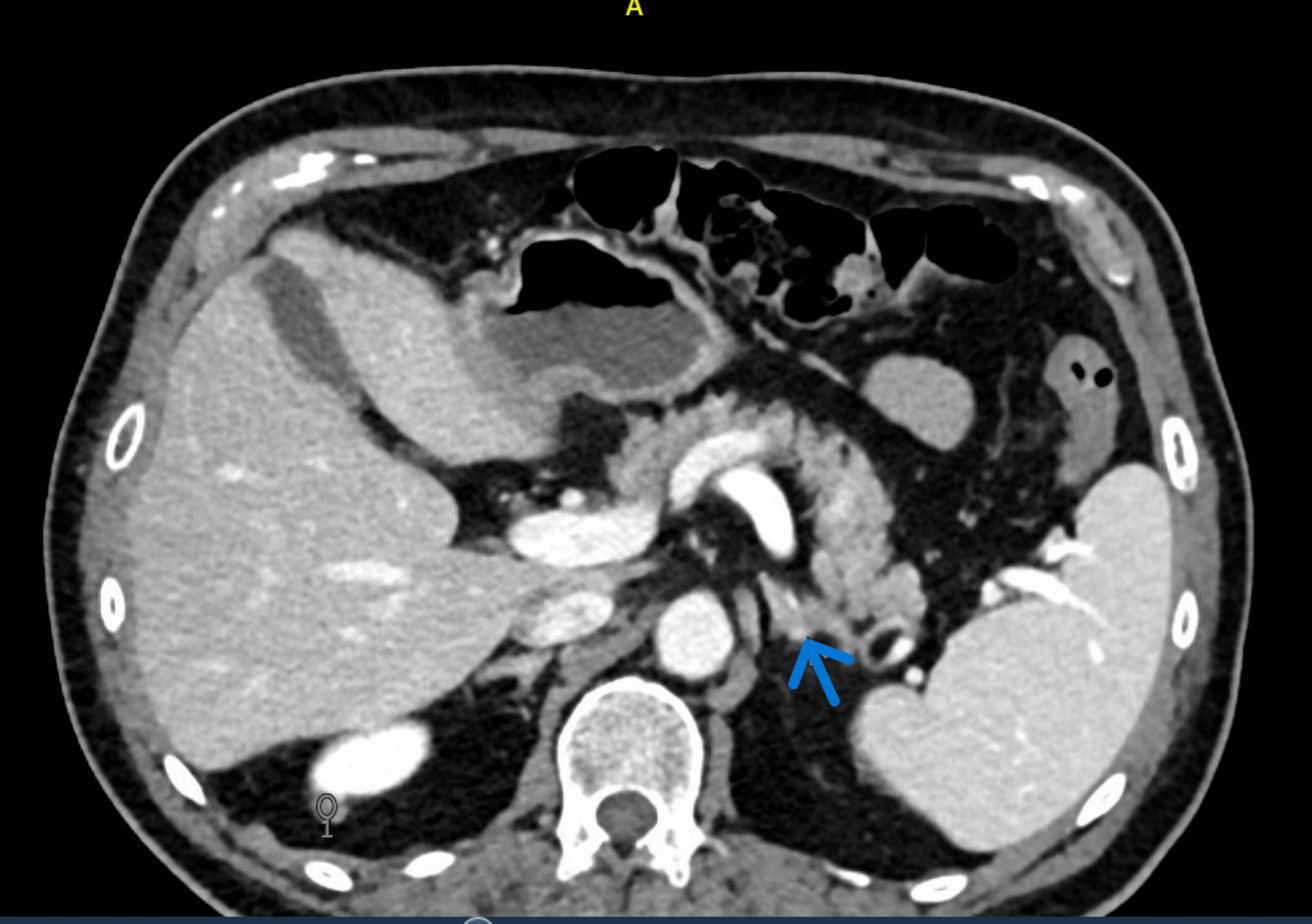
CT image of abdomen Left adrenal nodules observed in the CT image (blue arrow).
